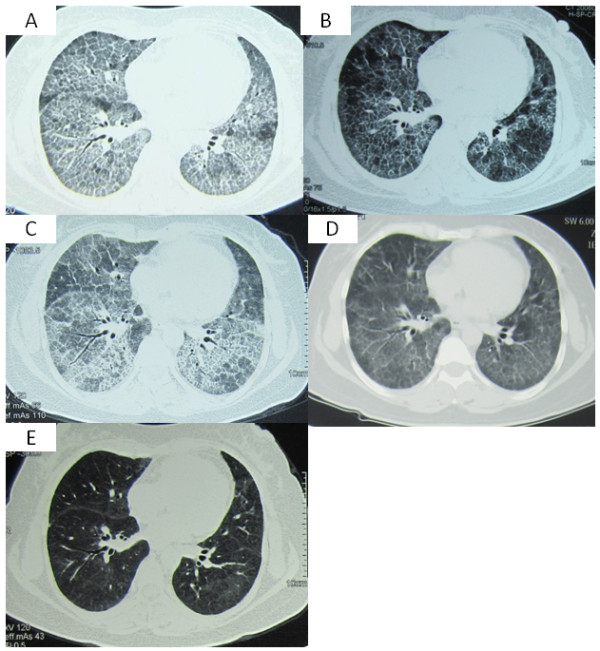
Series of her chest CT. A. The patient’s chest CT at the time of diagnosis of PAP. Her chest CT on Sep 10^th^ 2010 showed geographic ground-glass opacities combined with interlobular septal thickening (crazy paving) in both lungs. B. The patient’s chest CT after one month of the first WLL. On Oct 20^th^ 2010, about one month after the initial WLL, her repeated chest CT showed that the ground-glass opacities were diminished in both lungs. C. The patient’s chest CT during the the fourth refractory episode of PAP. On May 26^th^ 2012, the patient’s chest CT showed increased crazy paving in both lungs. D. The patient’s chest CT after the combination of WLL and 5 weeks of inhalation of GM-CSF. Her chest CT on Jul 8^th^ 2012 showed diminished shadows in both lungs. E. The patient’s chest CT after the combination of WLL and 15 weeks of inhalation of GM-CSF. There was significant improvement in her repeated chest CT on Sep 17^th^ 2012.
